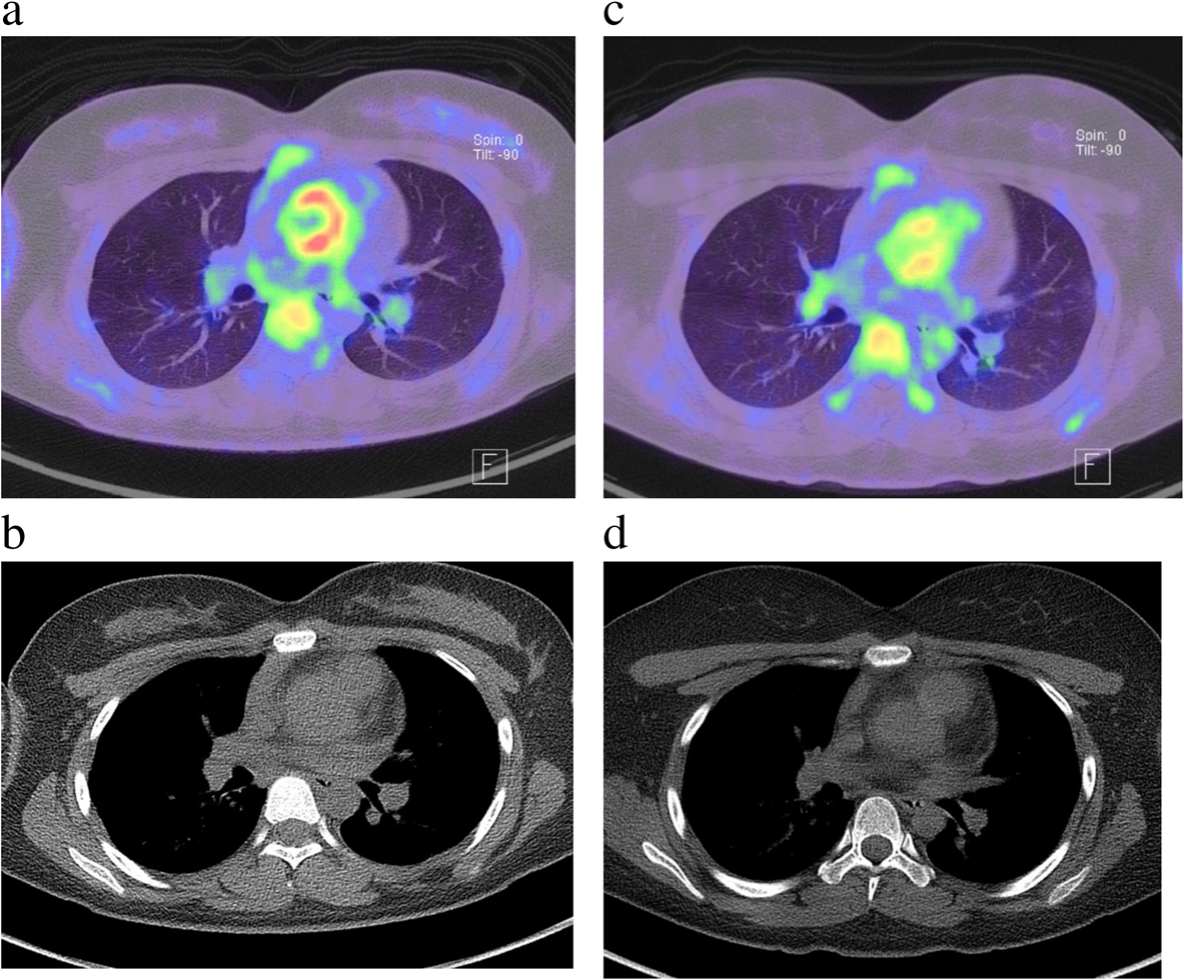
^18^F-fluorodeoxyglucose (FDG) positron emission tomography (PET)/computed tomography (CT). a, b. ^18^F-FDG PET/CT showed isolated inflammation of the aortic root on admission. The maximum standardized uptake value (max SUV) was 6.3. c, d. After immunosuppressive treatment, ^18^F-FDG PET/CT showed decreased ^8^F-FDG uptake in the aortic root. The max SUV was decreased to 4.3
